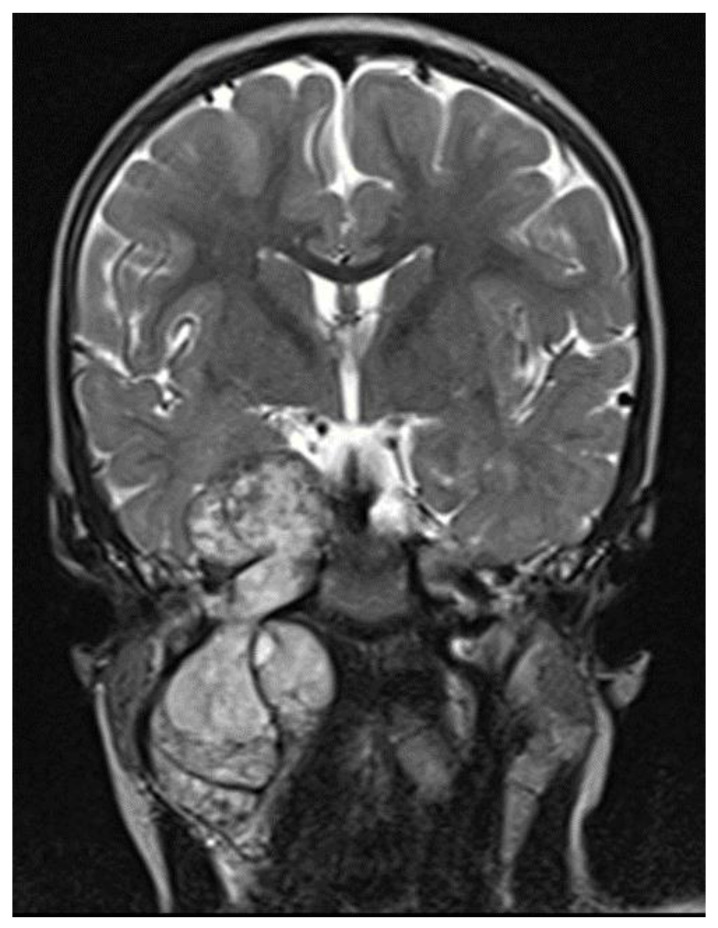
MRI T2 images, intratumor heterogeneity.
